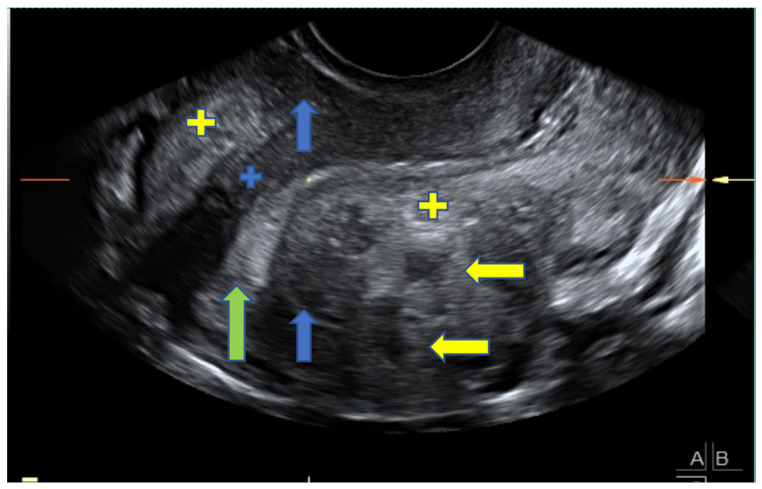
2D transvaginal longitudinal ultrasound image in a symptomatic patient, depicting the uterus with sonographic signs typical of adenomyosis: asymmetrical myometrial thickening (blue arrows), linear striations (blue plus sign), myometrial cysts (yellow arrows), hyperechoic islands (yellow plus sign), and an S-shaped endometrium (green arrow).
